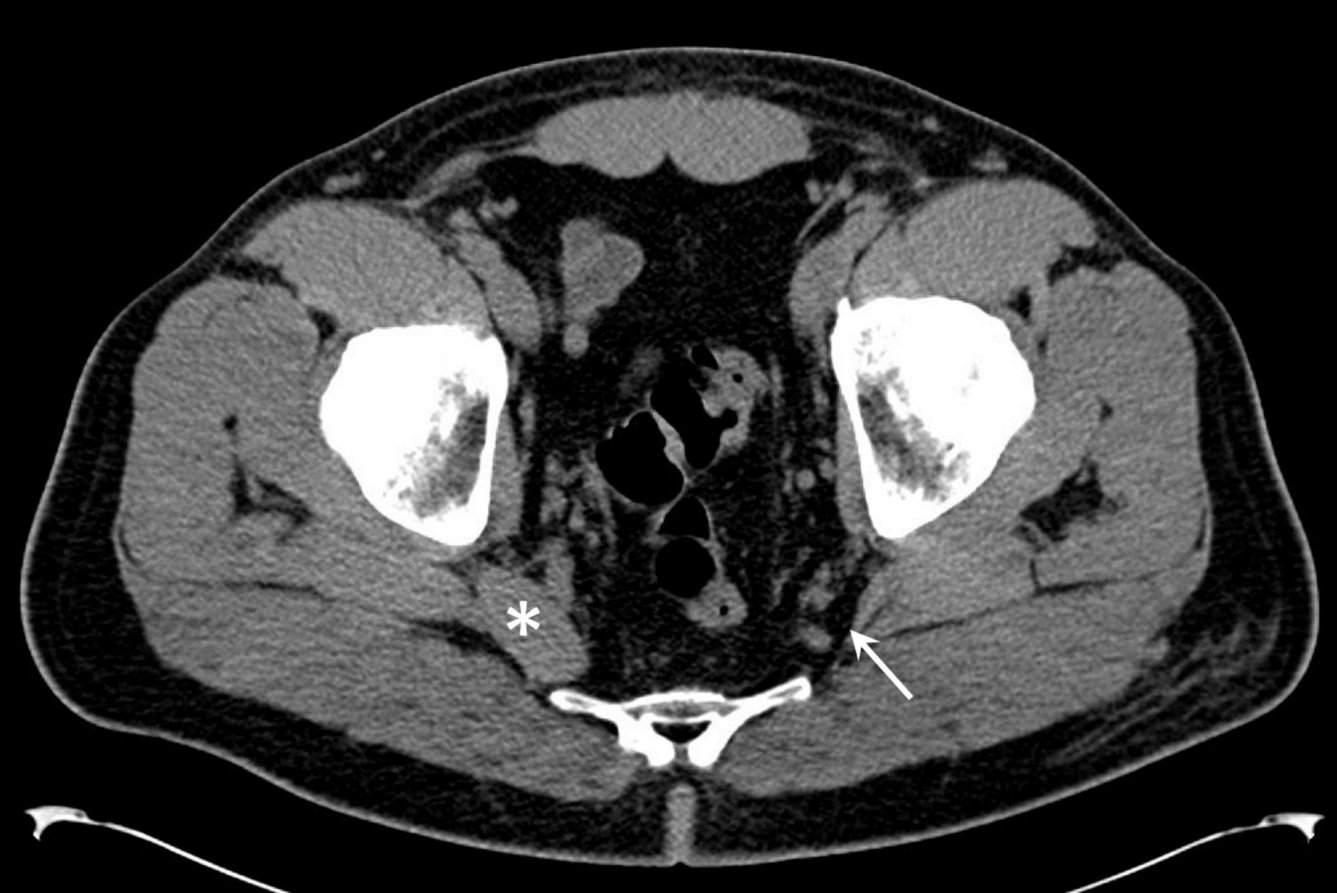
Computed tomography evaluation of the piriformis muscle. Axial computed tomography slice at the pelvic floor level shows absence of piriformis muscle (arrow). The right piriformis muscle is unremarkable (asterisk).
